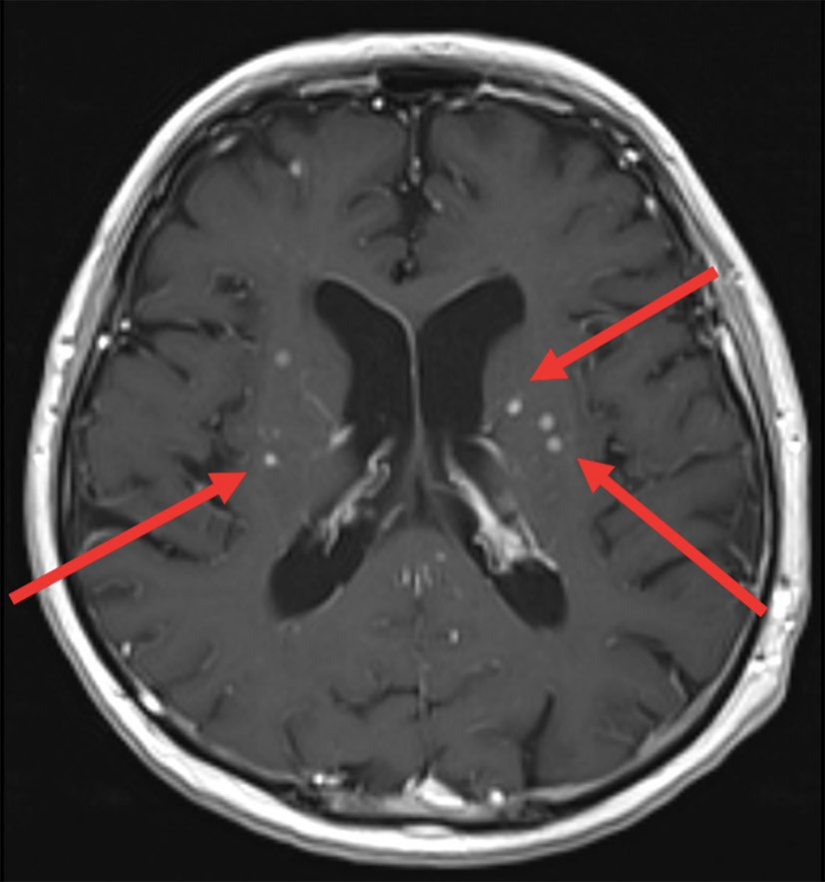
MRI of brain T1 (+ gadolinium) sequence, showing multiple micro-abscesses (hyperintensities pointed by the arrows)
